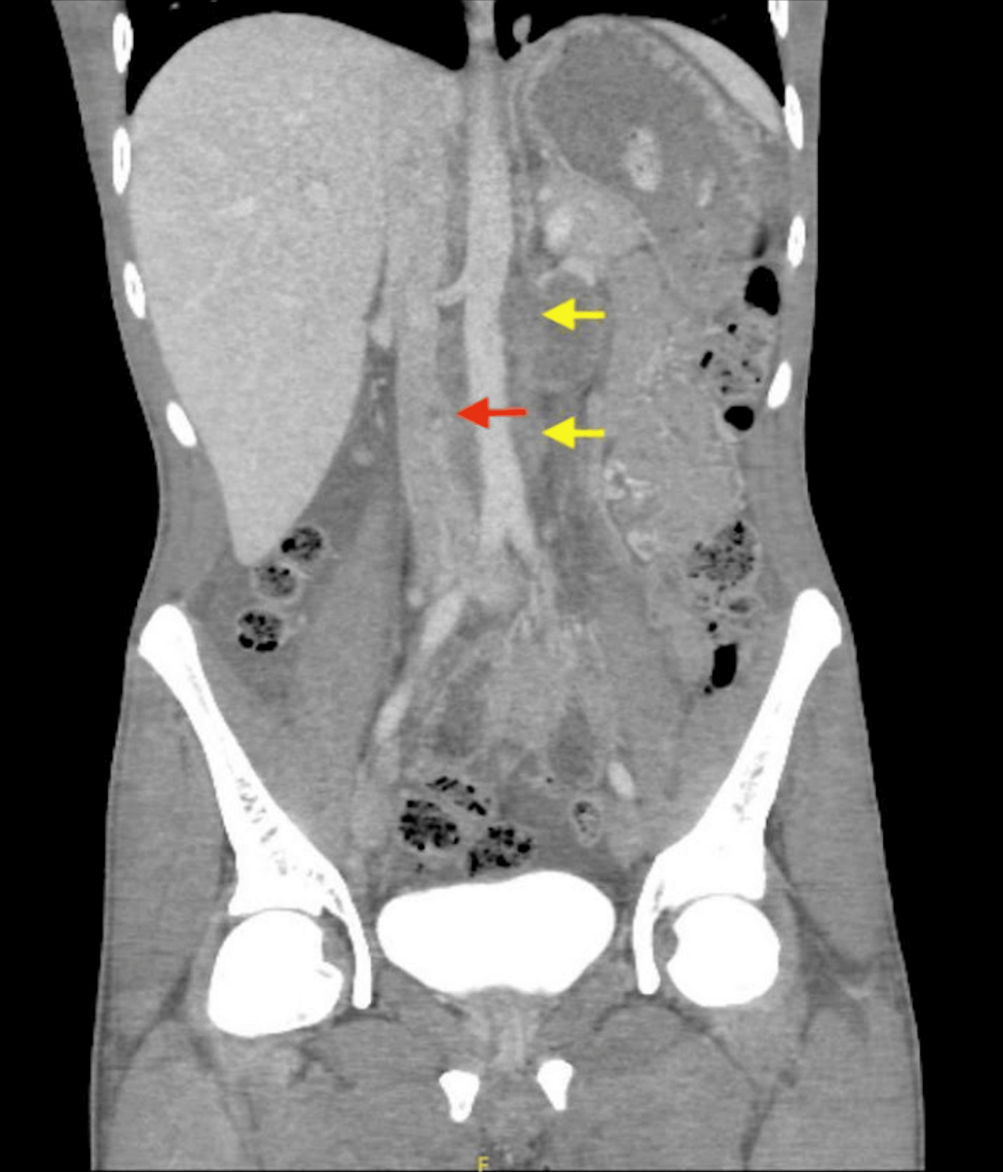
Coronal section of the CT scan of the abdomen The image demonstrated an IVC thrombus causing a filling defect measuring 8 mm, roughly 3.5 cm below the level of renal veins (indicated by the red arrow), with multiple para-aortic lymph node enlargement compressing the abdominal aorta (indicated by the yellow arrows) IVC: inferior vena cava; CT: computed tomography
